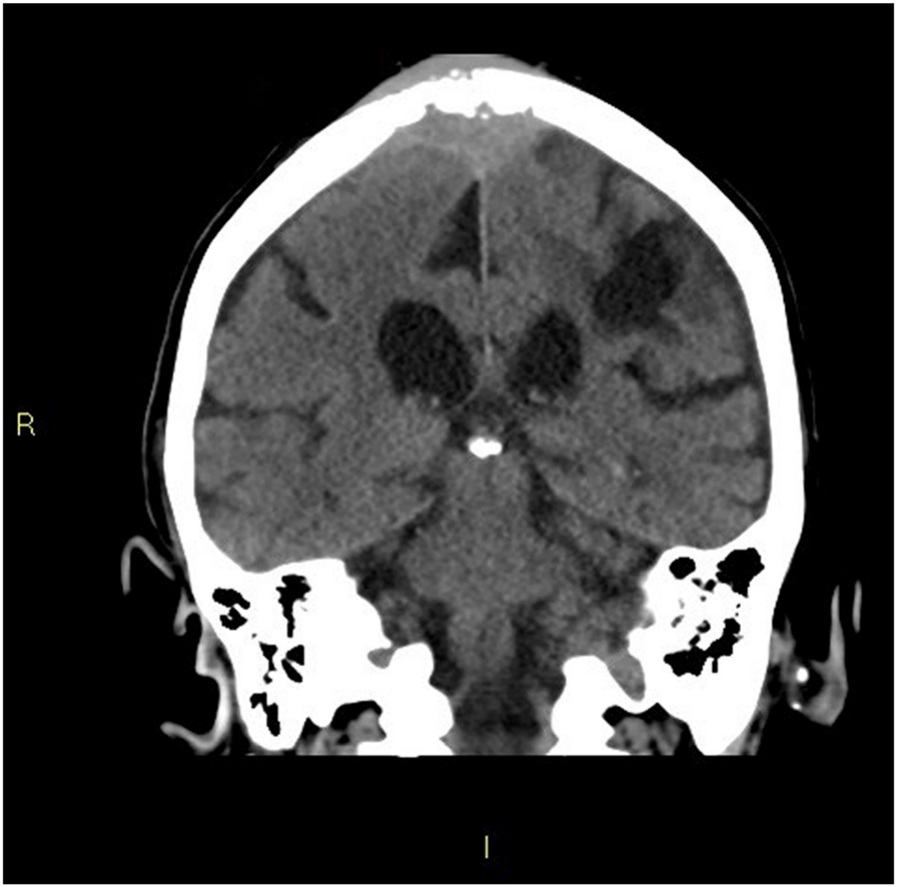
Chest X-ray. This is a posteroanterior chest X-ray taken of the patient at the time of diagnosis. It demonstrates the opacity in the left upper lobe of the lung consistent with lung cancer.
